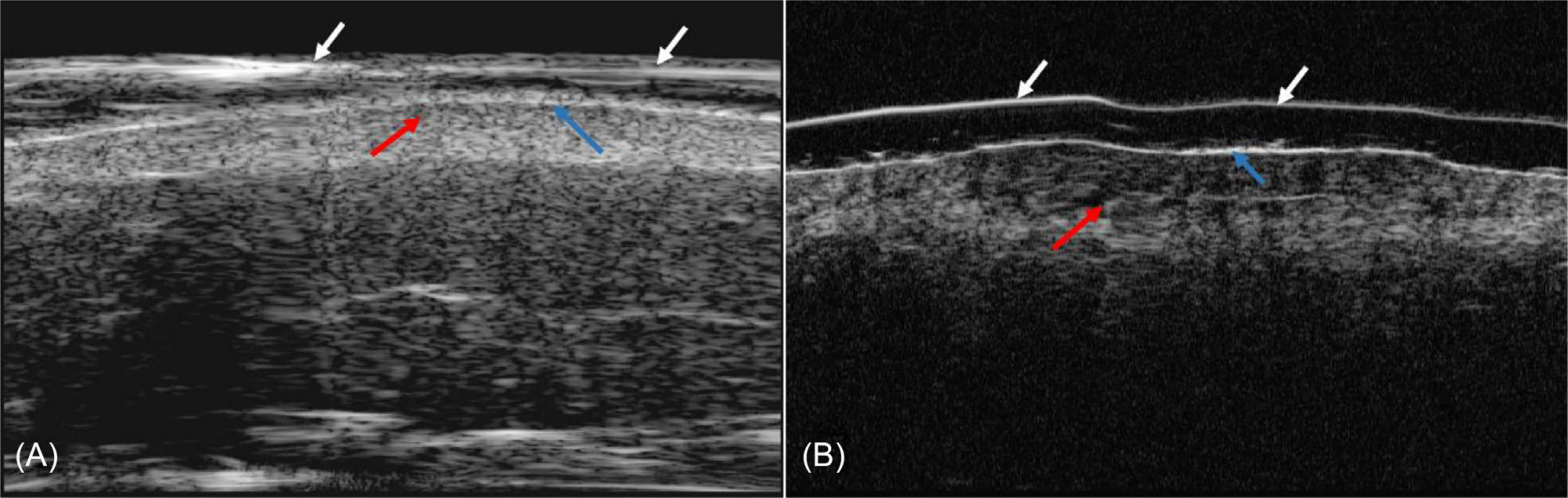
High‐frequency ultrasound examination revealed a hypoechoic zone at the dermal‐epidermal junction and superficial dermis (red arrows),thickened epidermis (blue arrows). Foil membrane (white arrows). (A: 20 Hz, B: 50 Hz).
